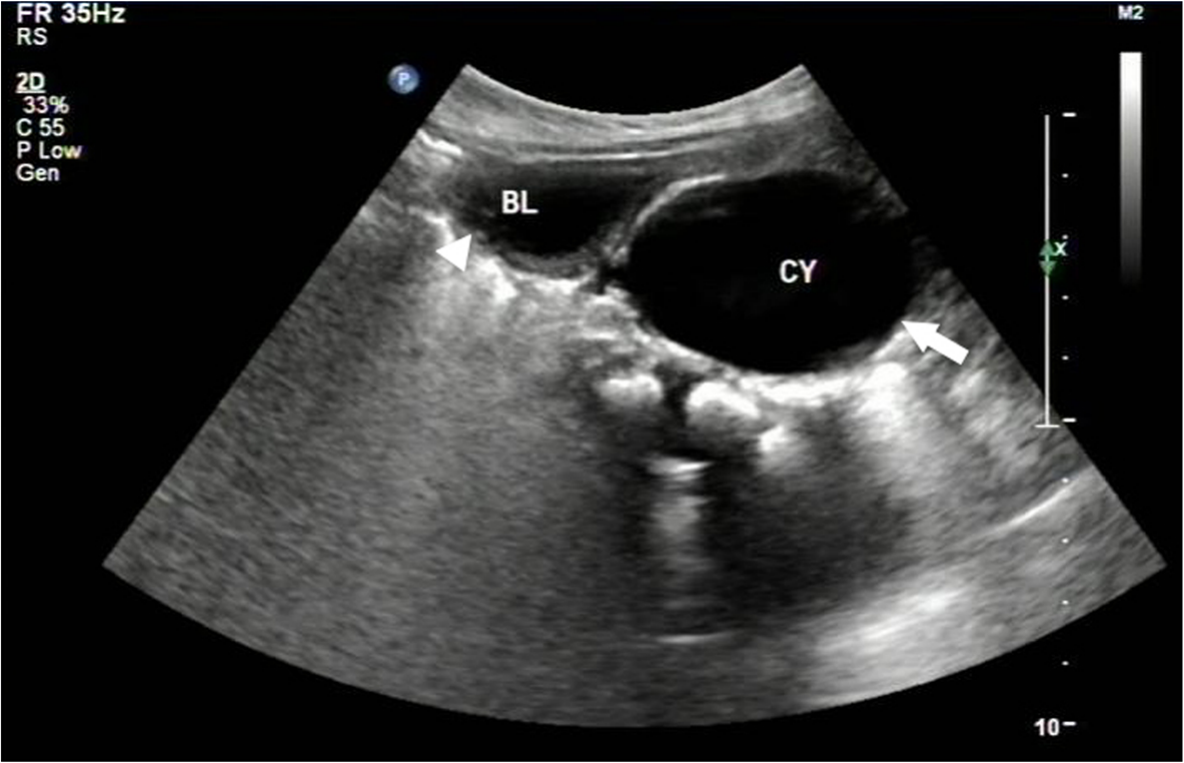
Transverse ultrasound of the pelvis shows a large utricle in the midline (white arrow), posterior to the urinary bladder (white arrowhead)
